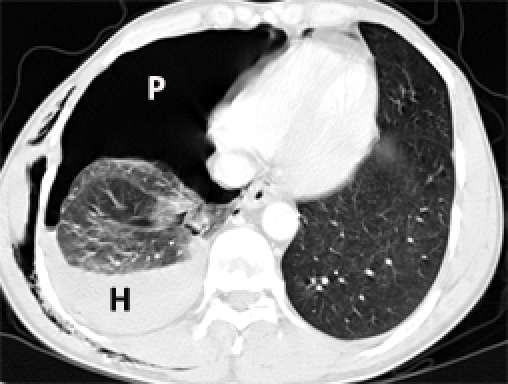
Thoracic CT. P – pneumothorax. H – hemothorax
